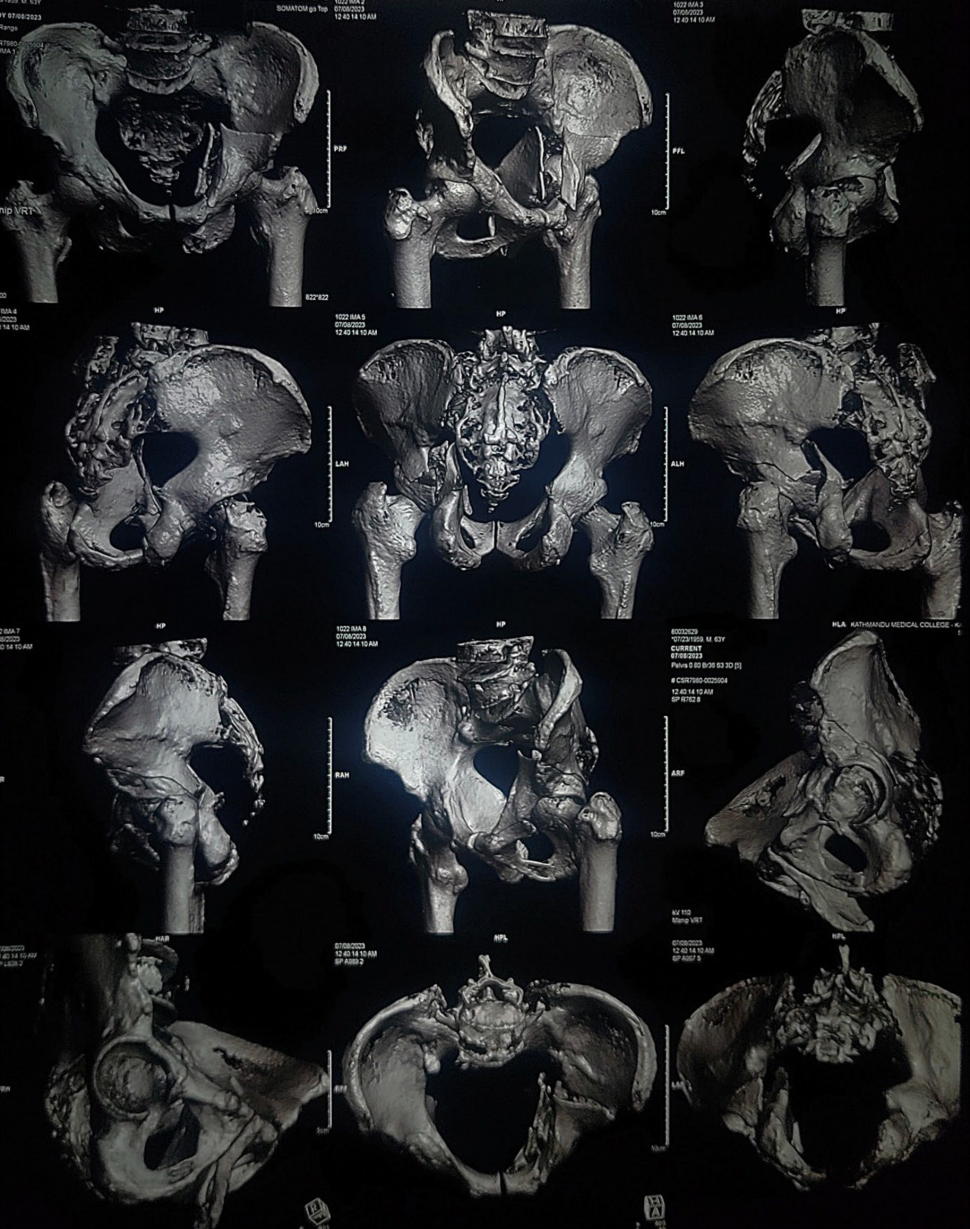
Nonenhanced computed tomography (NECT) of pelvis with 3D reconstruction.
